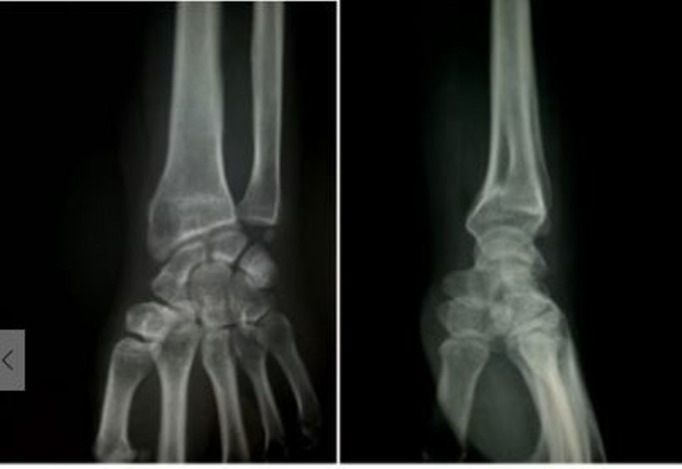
Anteroposterior and lateral x-ray of wrist after six months follow-up showing good union
